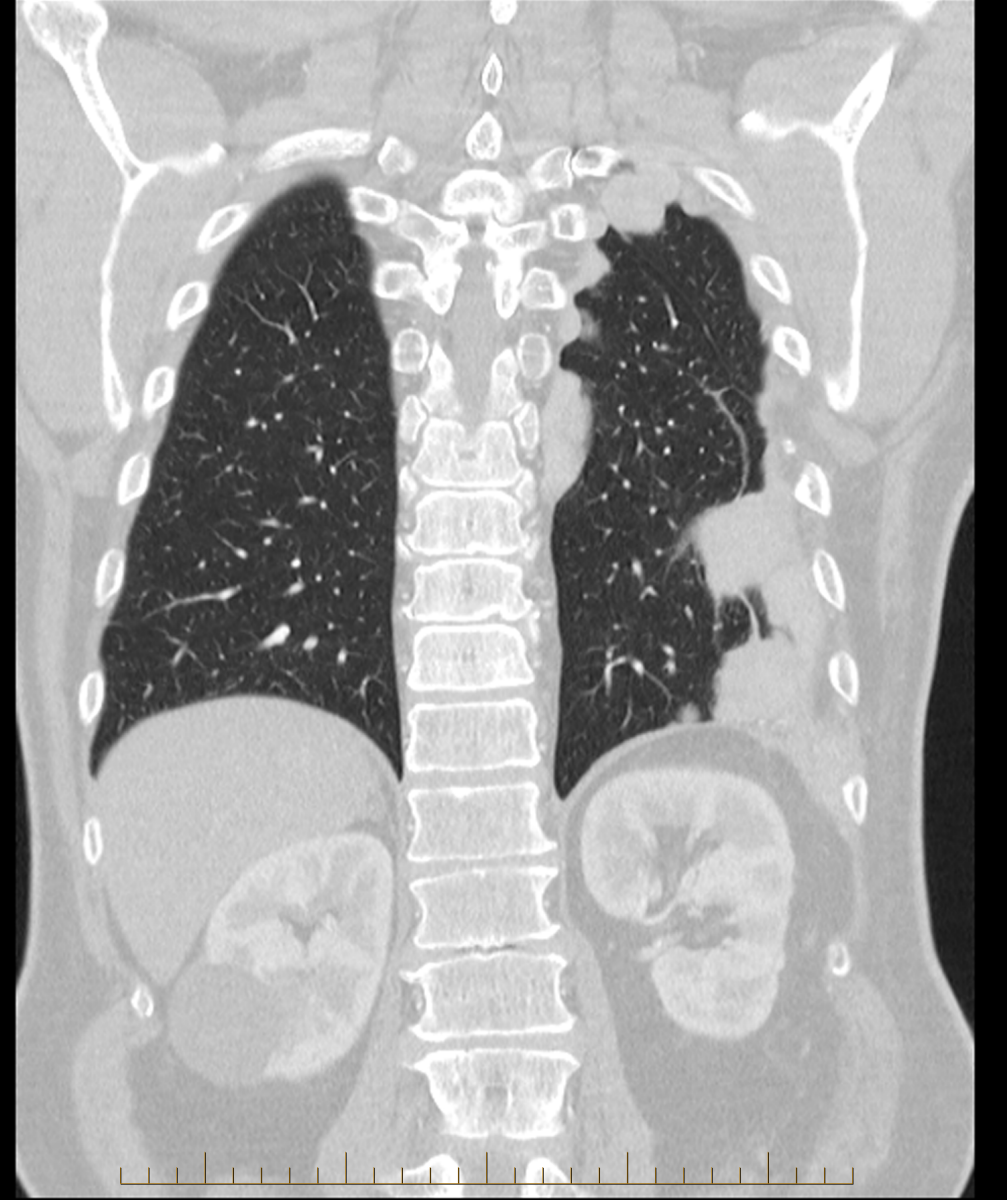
A coronal slice from a computed tomography scan of the chest showing lobulated pleural based masses with some round atelectasis.
